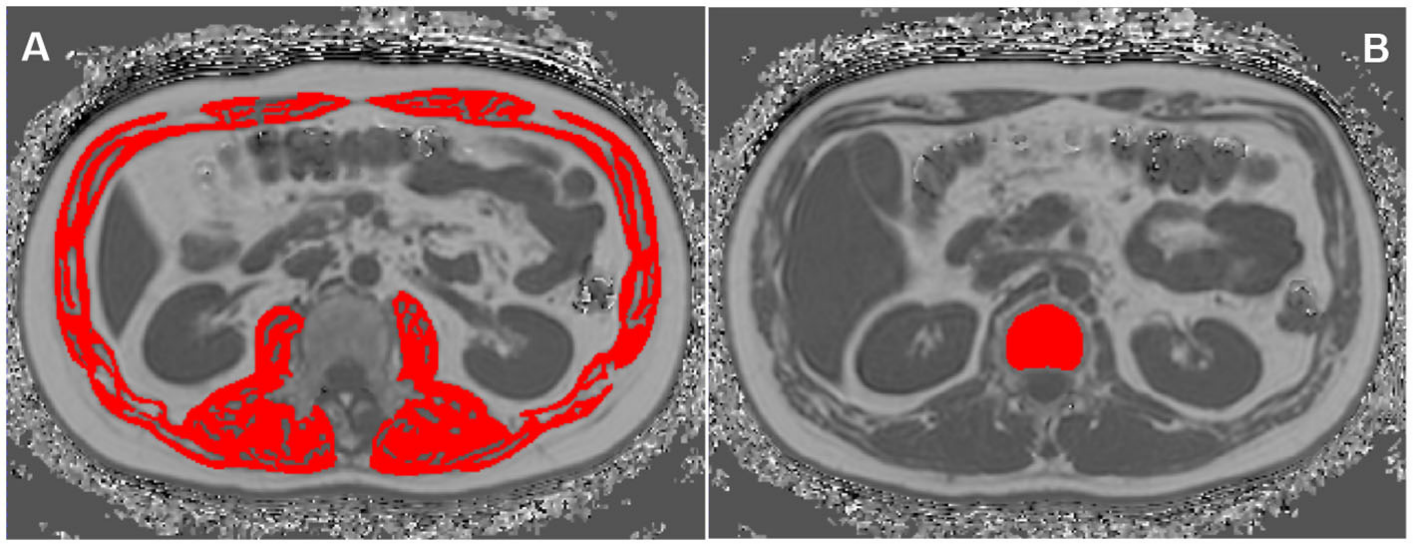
Region of interests (ROIs) of abdominal muscle (AM) (A) and vertebral bone marrow adipose tissue (BMAT) (B) on the MRI fat fraction (FF) mapping.
